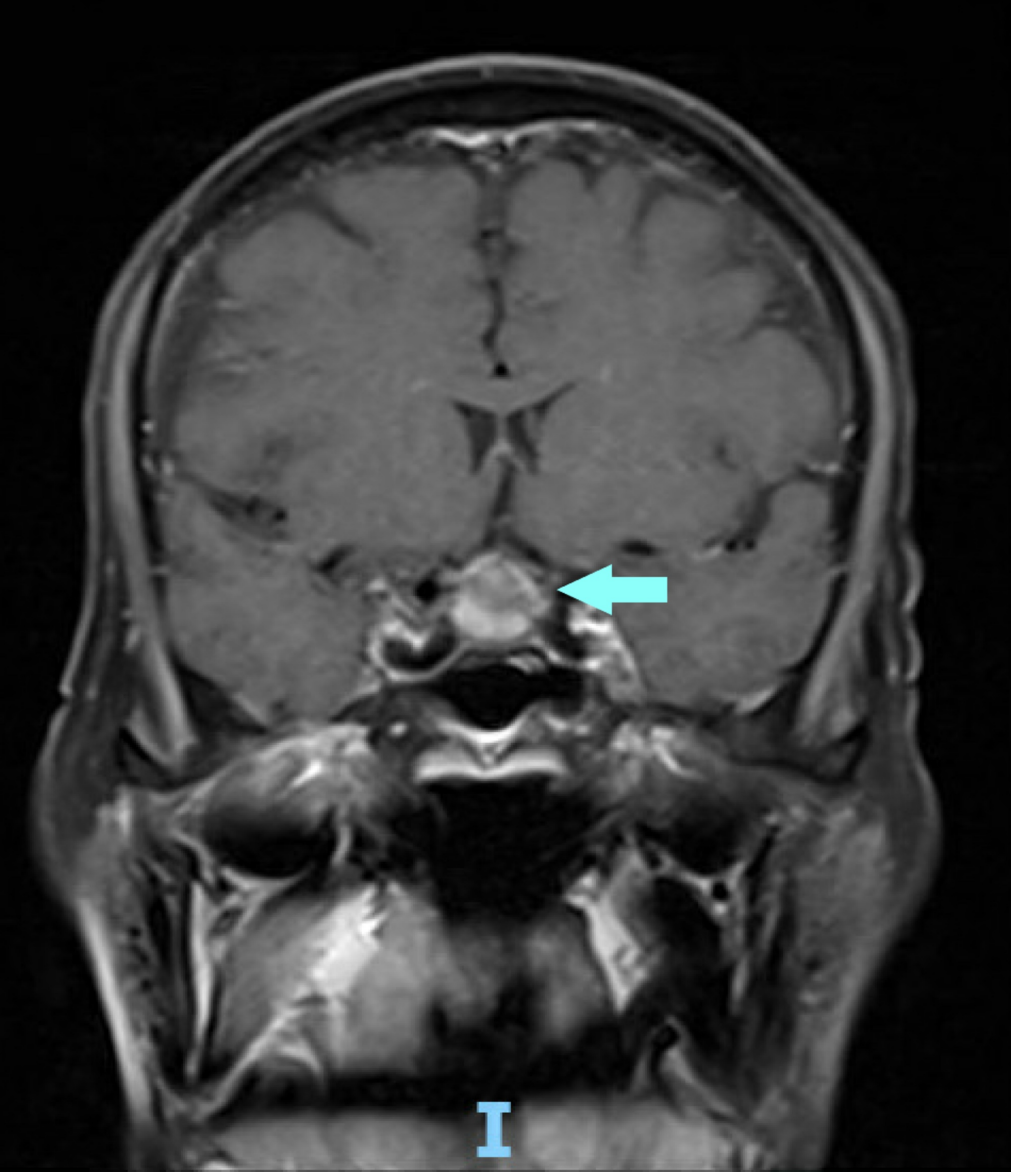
Magnetic resonance imaging showing pituitary macroadenoma in coronal view
